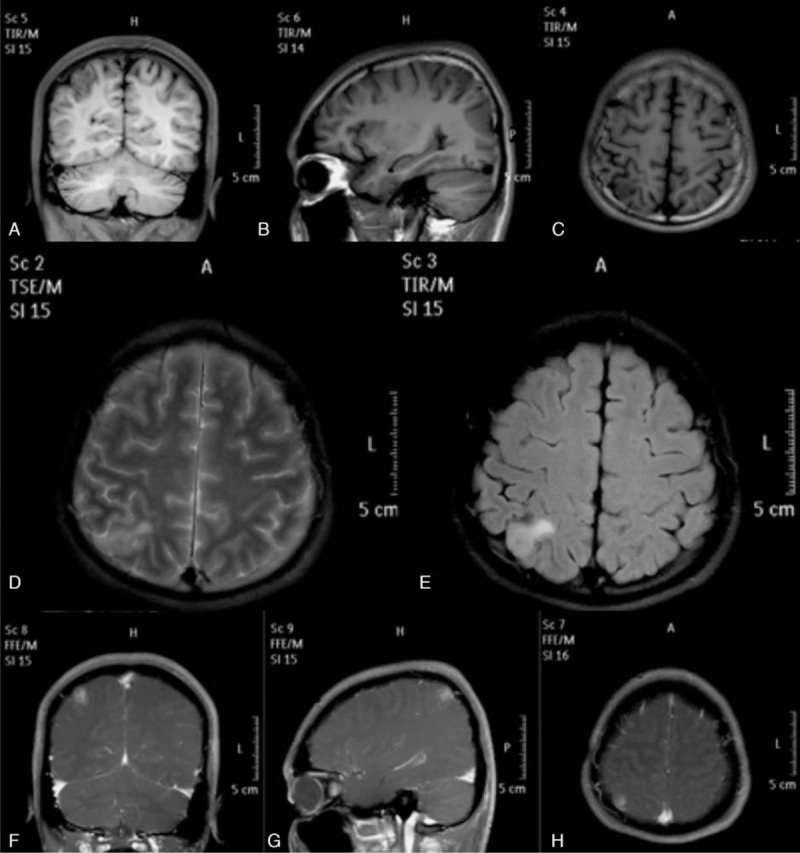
Preoperative MRI in Case 1. Coronal (A), sagittal (B), and axial (C) T1-weighted imaging showed a hypointense lesion in the right parietal lobe. The lesion was hyperintense on T2-weighted imaging (D) and FLAIR imaging (E). Coronal (F), sagittal (G), and axial (H) contrast T1-weighted imaging showed the lesion was heterogeneously enhanced.
